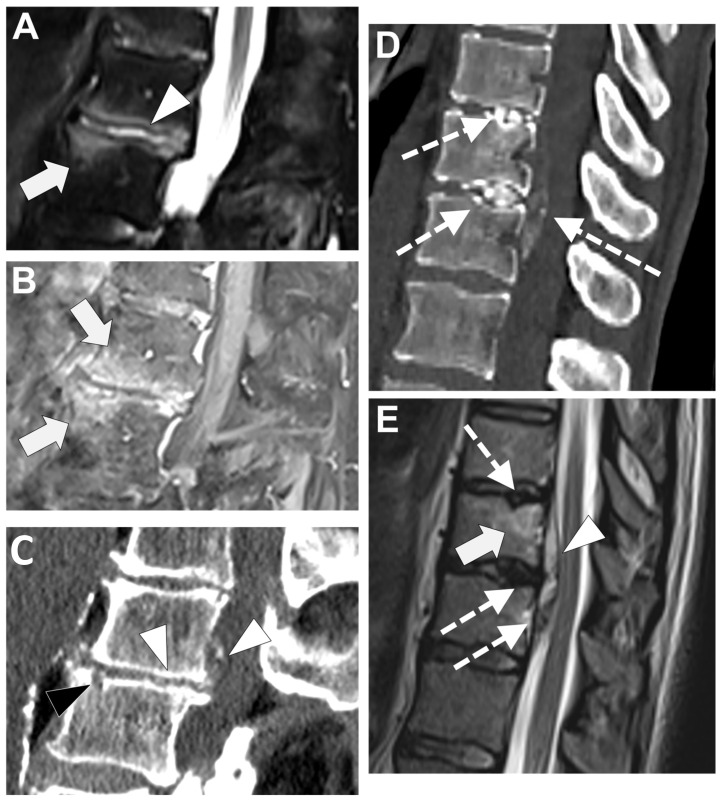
Examples of microcristalline spinal disorders. A 78-year-old male presented with acute inflammatory pain of the upper lumbar spine. An MRI was performed with (A) STIR T2-weighted imaging (WI) and (B) fat sat T1-WI after gadolinium chelates injection, followed by a CT scan (C). It shows a linear high signal (SI) of a narrowed disk (white arrowhead) on STIR T2-WI with multiple small powdery calcifications on CT-scan, typical of chondrocalcinosis. It was associated with subchondral edema (white arrows) and a small endplate erosion (black arrowhead). A 35-year-old woman presented to the emergency for acute and intense dorsal pain. On CT-scan (D), gross and dense calcifications of two adjacent disks were observed (typical of hydroxyapatite) with a migration in the anterior epidural space (dashed white arrow). On T2-WI (E), the low T2-SI of the calcification can be observed (dashed white arrows), as well as a subtle edema of the posterior vertebral corner (white arrow) and the thickening of the epidural space (white arrowhead).
